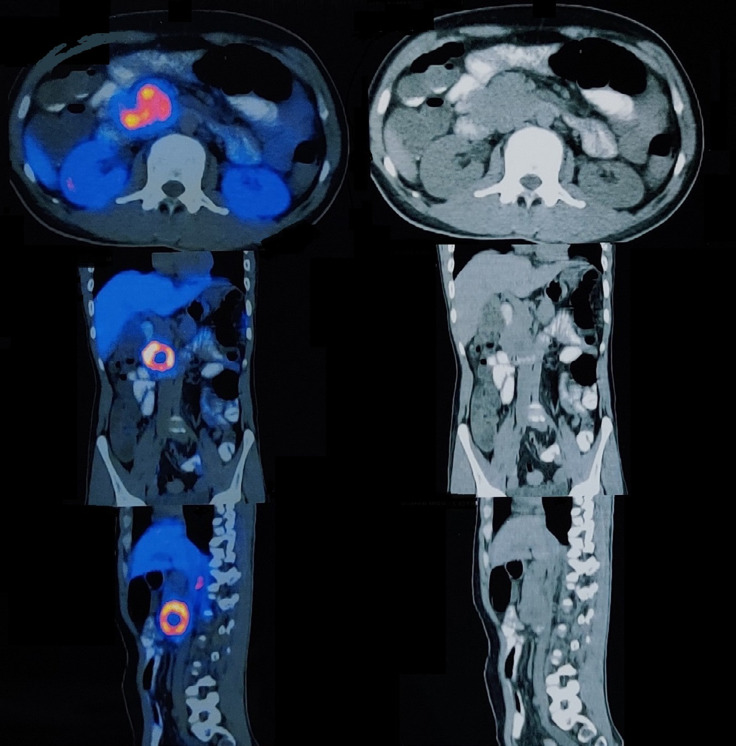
Axial, coronal, and sagittal section images of ^68^Ga DOTANOC PET CT scan showing enhancing lesion in the head of pancreas
